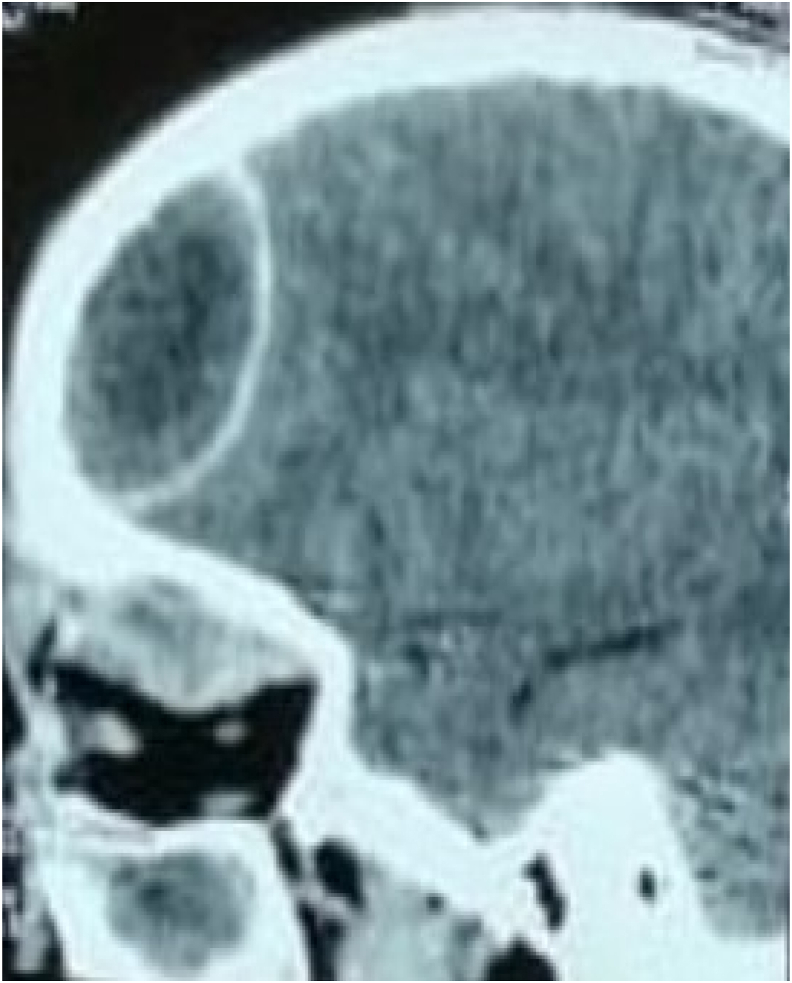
CT scan imaging showing left frontal epidural abscess with enhancement of the lesion's rim after addition of contrast material with an orbital abscess.
